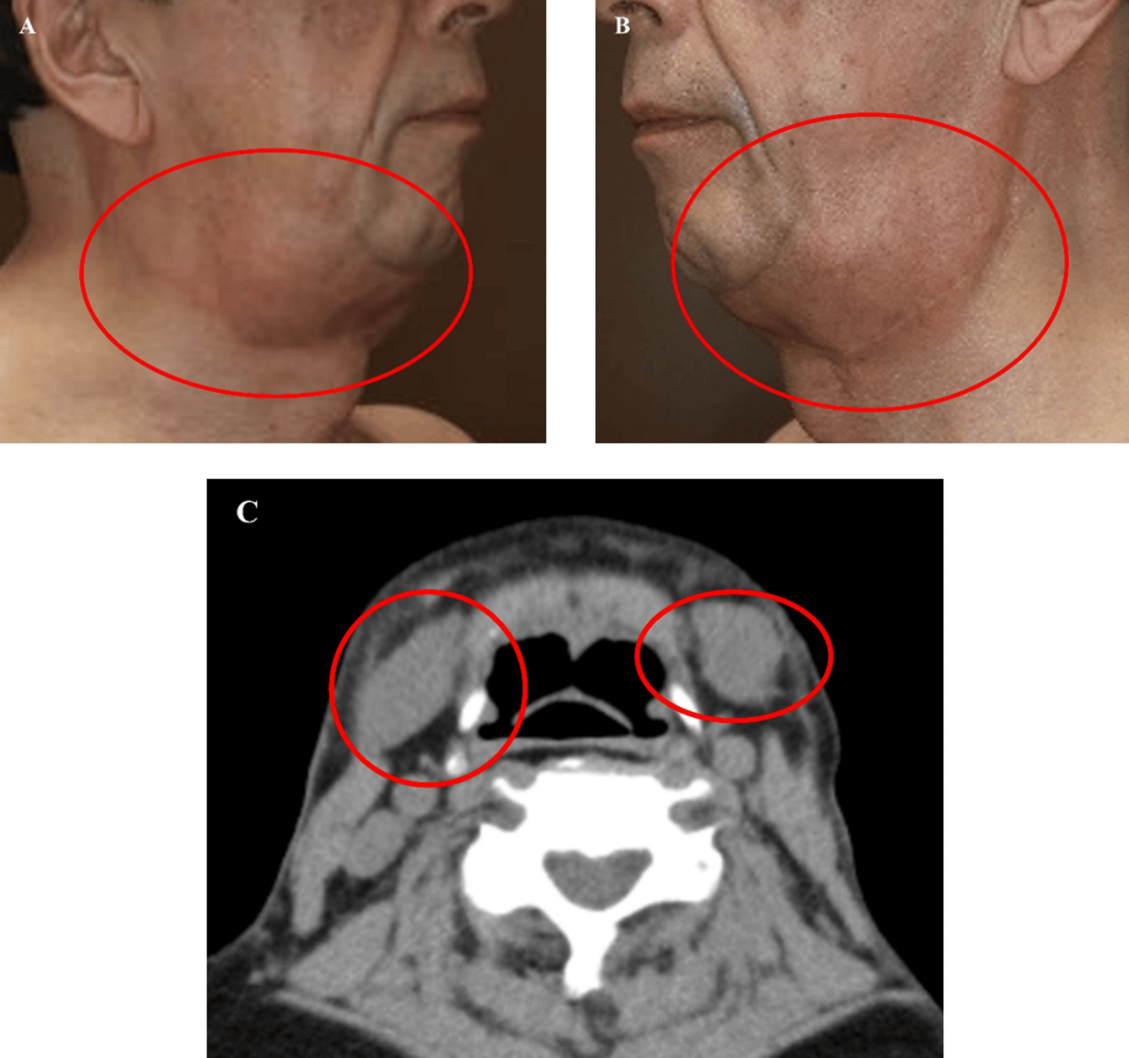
Submandibular gland imaging Clinical and radiological evaluation demonstrating bilateral submandibular gland enlargement contributing to residual submental fullness. (A, B) Preoperative clinical photographs showing gland prominence on the left and right sides, respectively. (C) Axial CT scan highlighting bilateral submandibular gland hypertrophy (circled in red).
